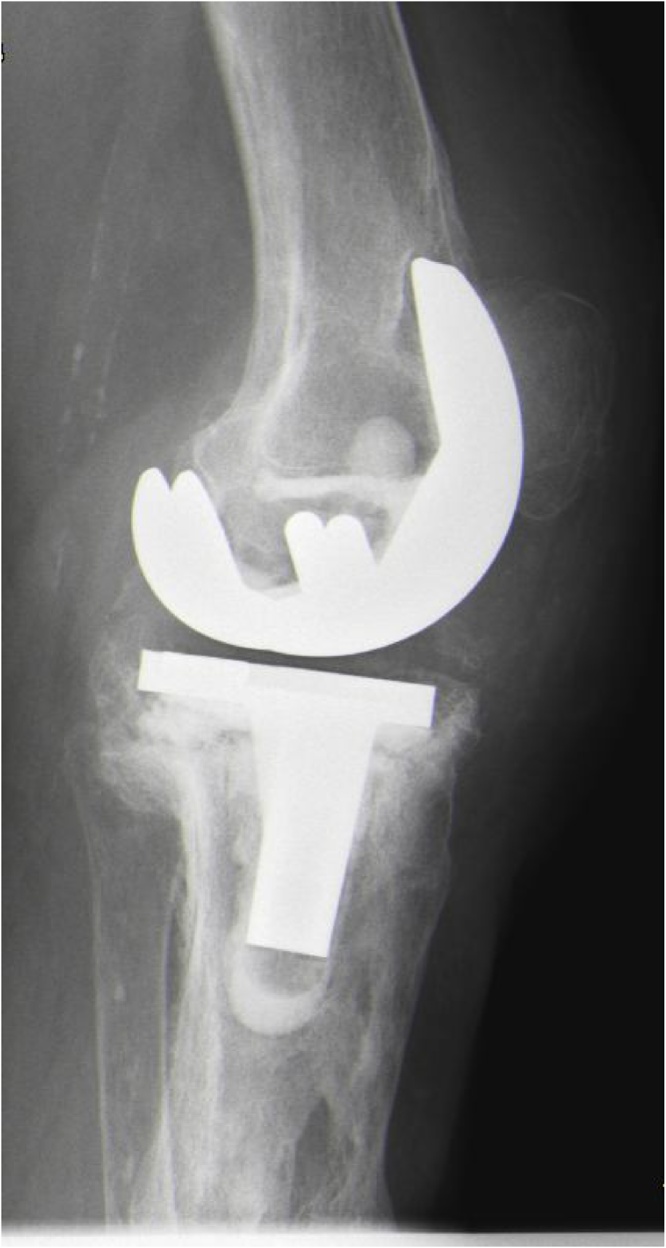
Lateral x-ray view of the total knee arthroplasty of the left knee. Extensive bone destruction is present in the proximal tibia. Loosening signs of the femoral component are present.
